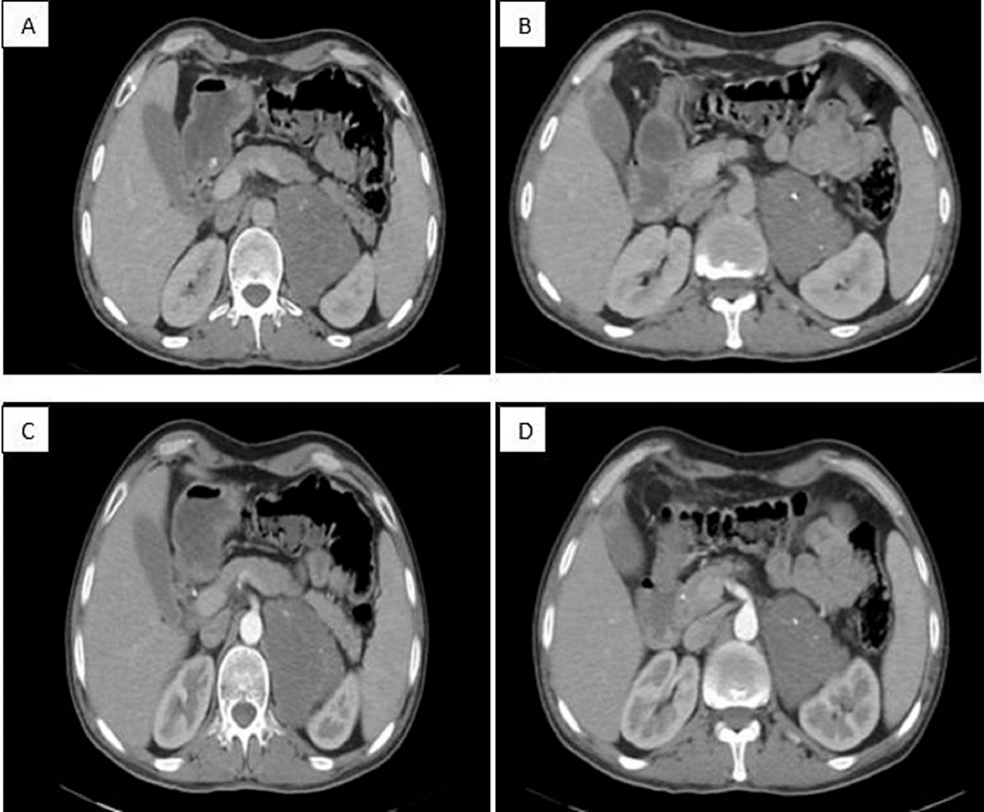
Cross-sectional CT scan C- (A, B), C+ (C,D) showing the triangular left adrenal mass with calcification.
